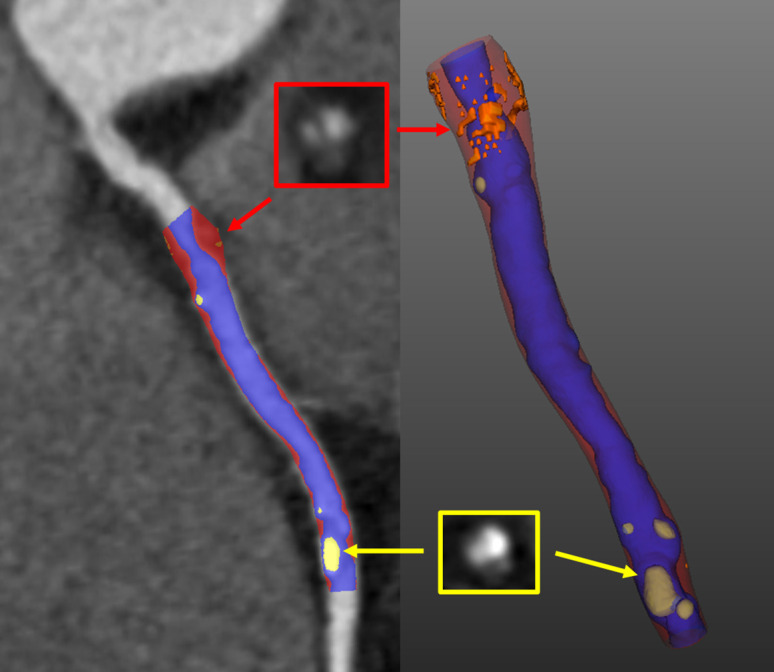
Computed tomography coronary angiography plaque analysis
